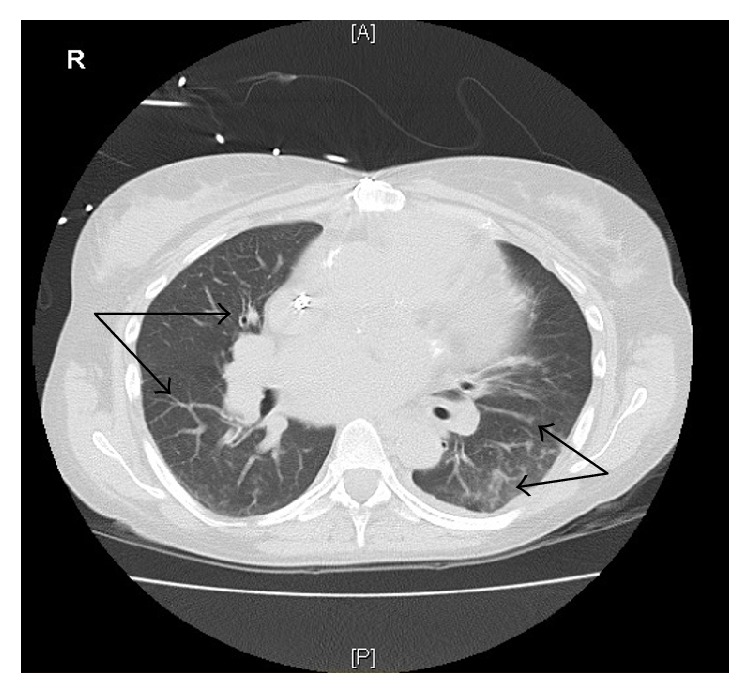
CT scan of the chest showing bilateral lungs ground-glass and tree-in-bud opacities.
